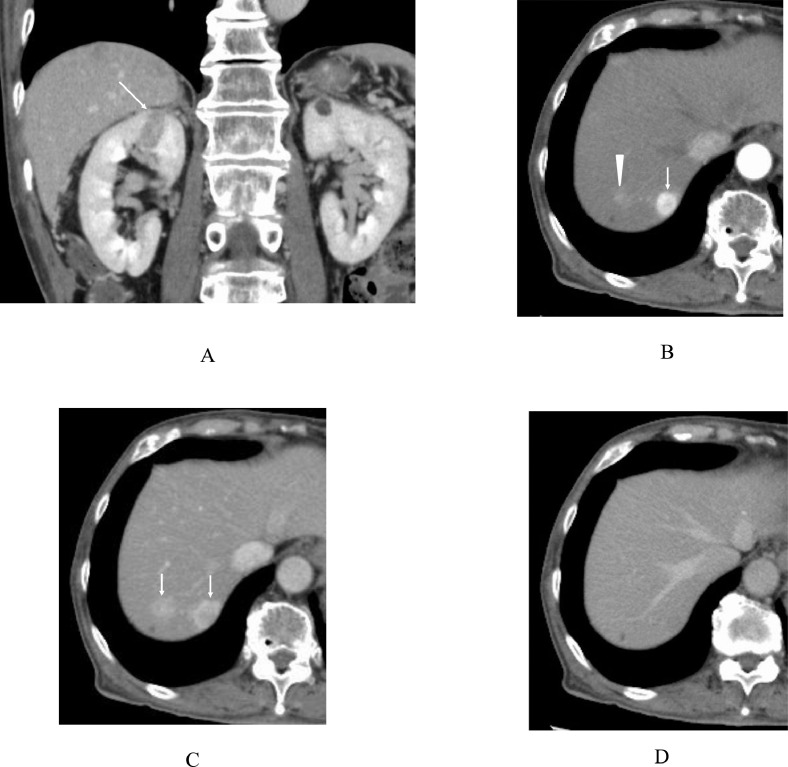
Enhanced computed tomography (CT) findings. (A) Coronal view of the enhanced CT showed an oval mass with slight enhancement in the right kidney (arrow). (B) Axial view of the enhanced CT, an arterial phase, showed round (arrow) and obscured (arrowhead) tumors with rapid enhancement in the liver. (C) Axial view of the enhanced CT, a portal phase, showed a marked washout in the liver tumors (arrows). (D) Axial view of the enhanced C, a late phase, showed complete washout in the liver tumors.
